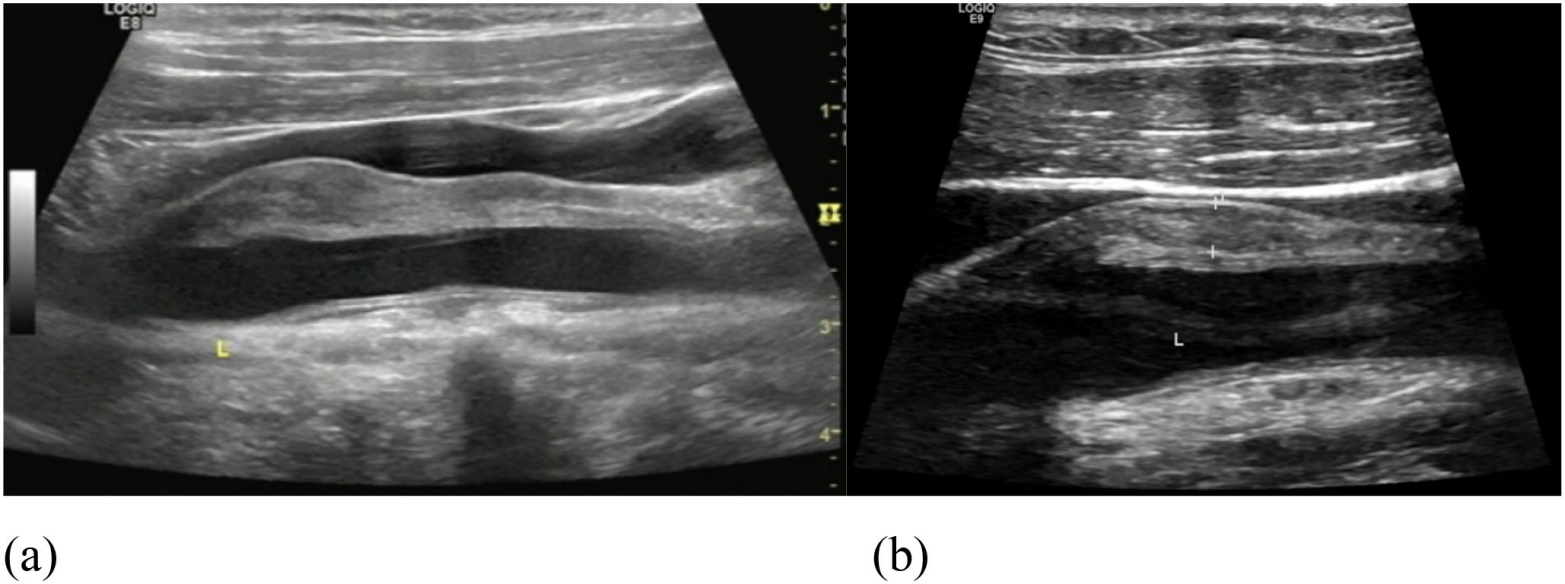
(a) Ultrasound before treatment showed a hypoechoic mass around the left common carotid artery. (b) Post-treatment ultrasound demonstrated reduction in the size of the hypoechoic mass surrounding the left common carotid artery.
